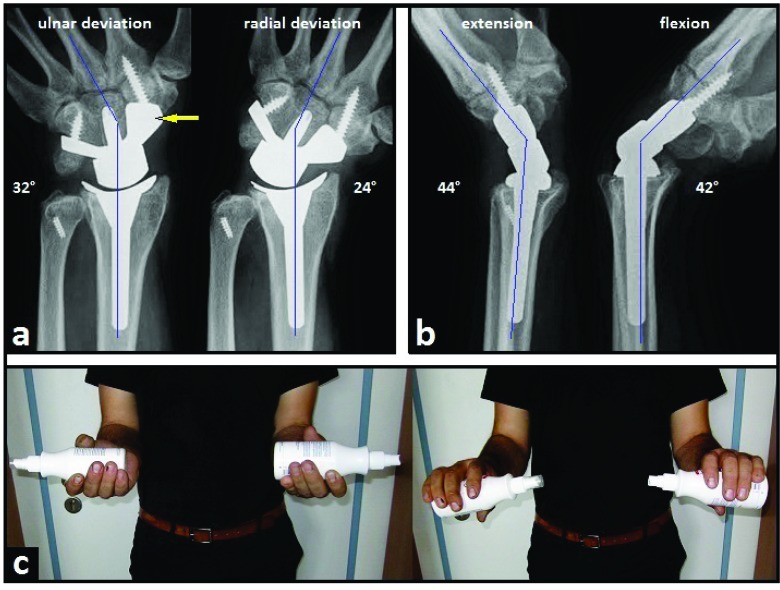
(Case Presentation, 1-year follow-up): (a) PA radiographs in terms of terminal ranges of motion showing unchanged correct positioning of TWA without implant loosening or subsidence or impingement or instability, note that the entire scaphoid bone was excised and a carpal plate utilizing a scaphoid augment (arrow) was used that allows its sufficient support onto the base of trapez bone without the necessity of fusion between the distal part of scaphoid bone to the surrounding carpal bones; (b) Lateral radiographs in terms of terminal ranges of motion showing no instability of implant; (c) Clinical photographs demonstrating 90° supination and 90° pronation of both forearms.
